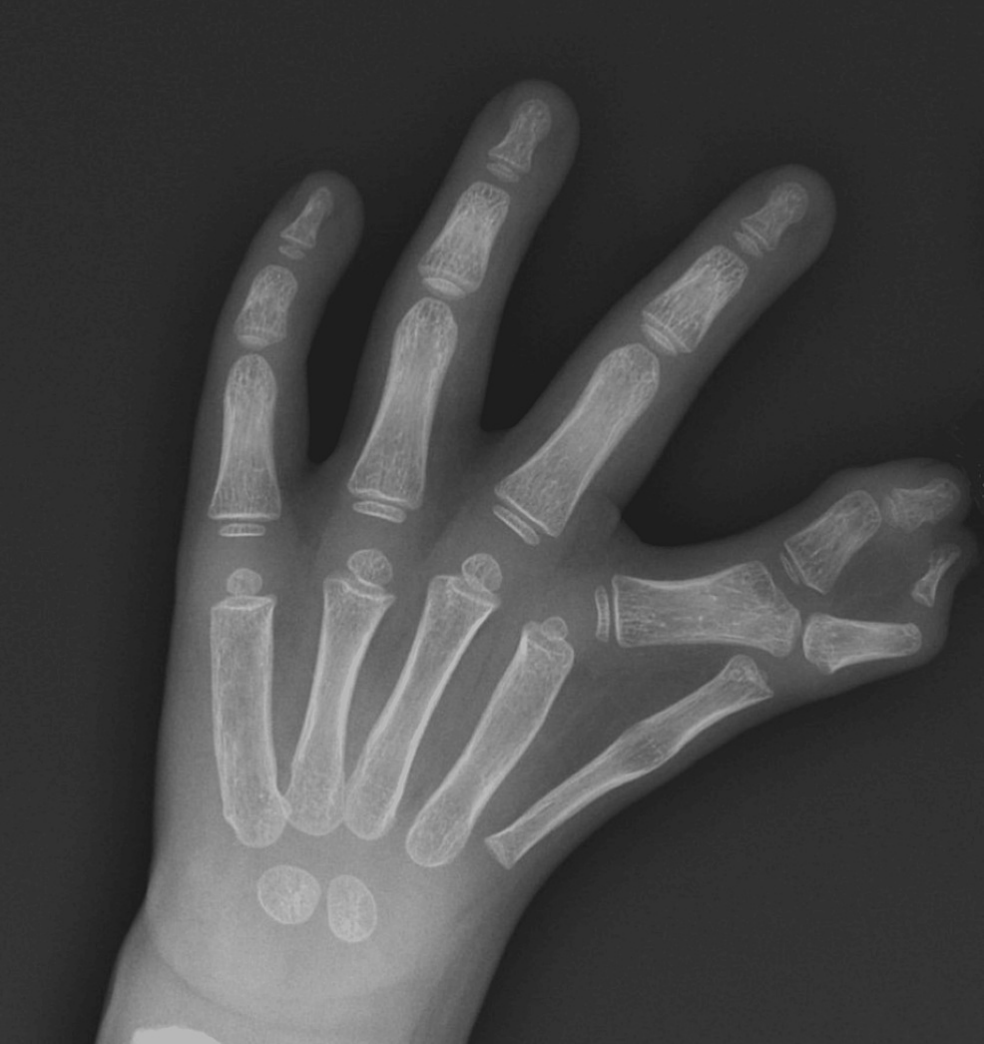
Radiograph of the left hand
